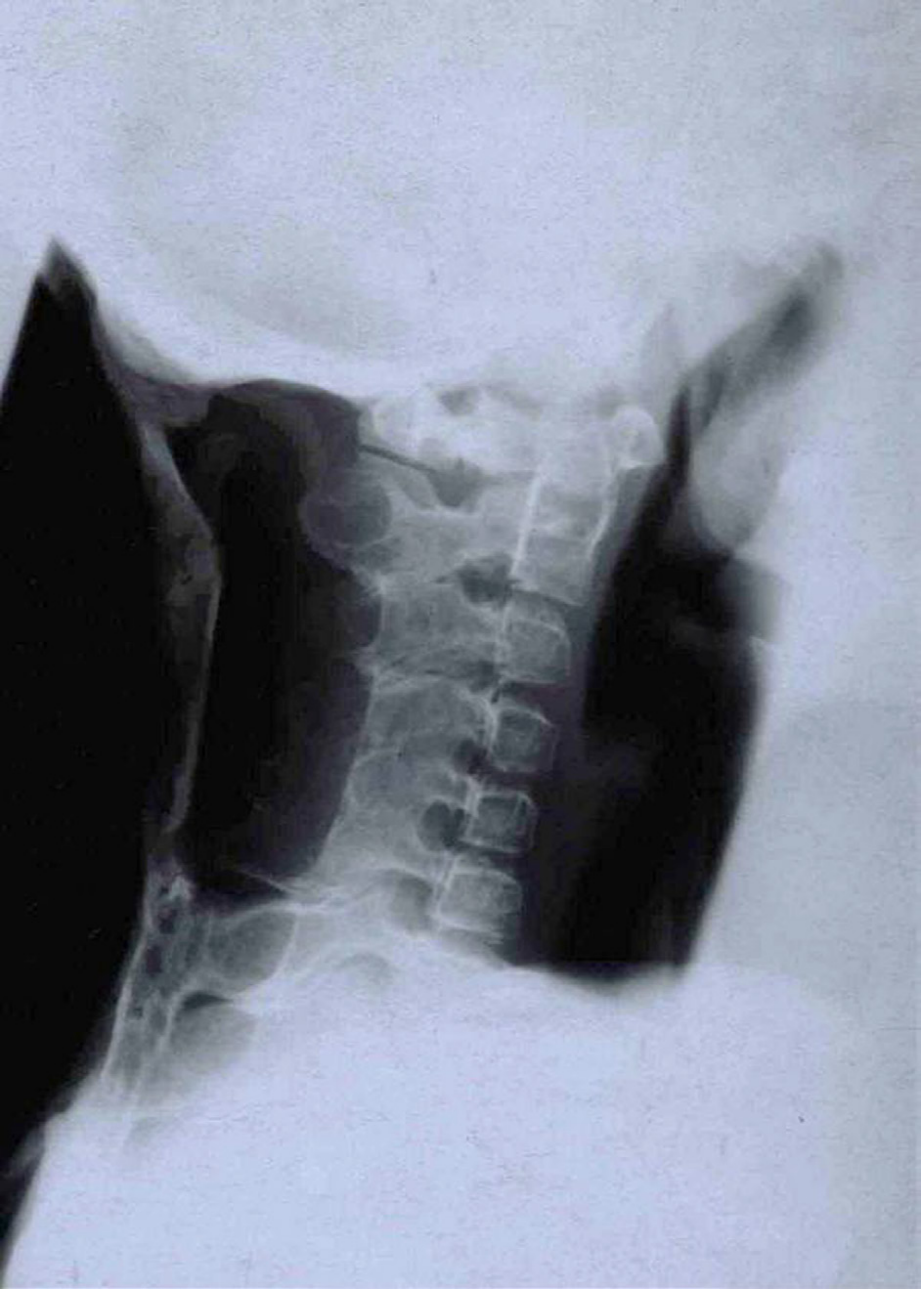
Lateral radiography of the neck showed ectopic ossification in the trapezius muscle.
